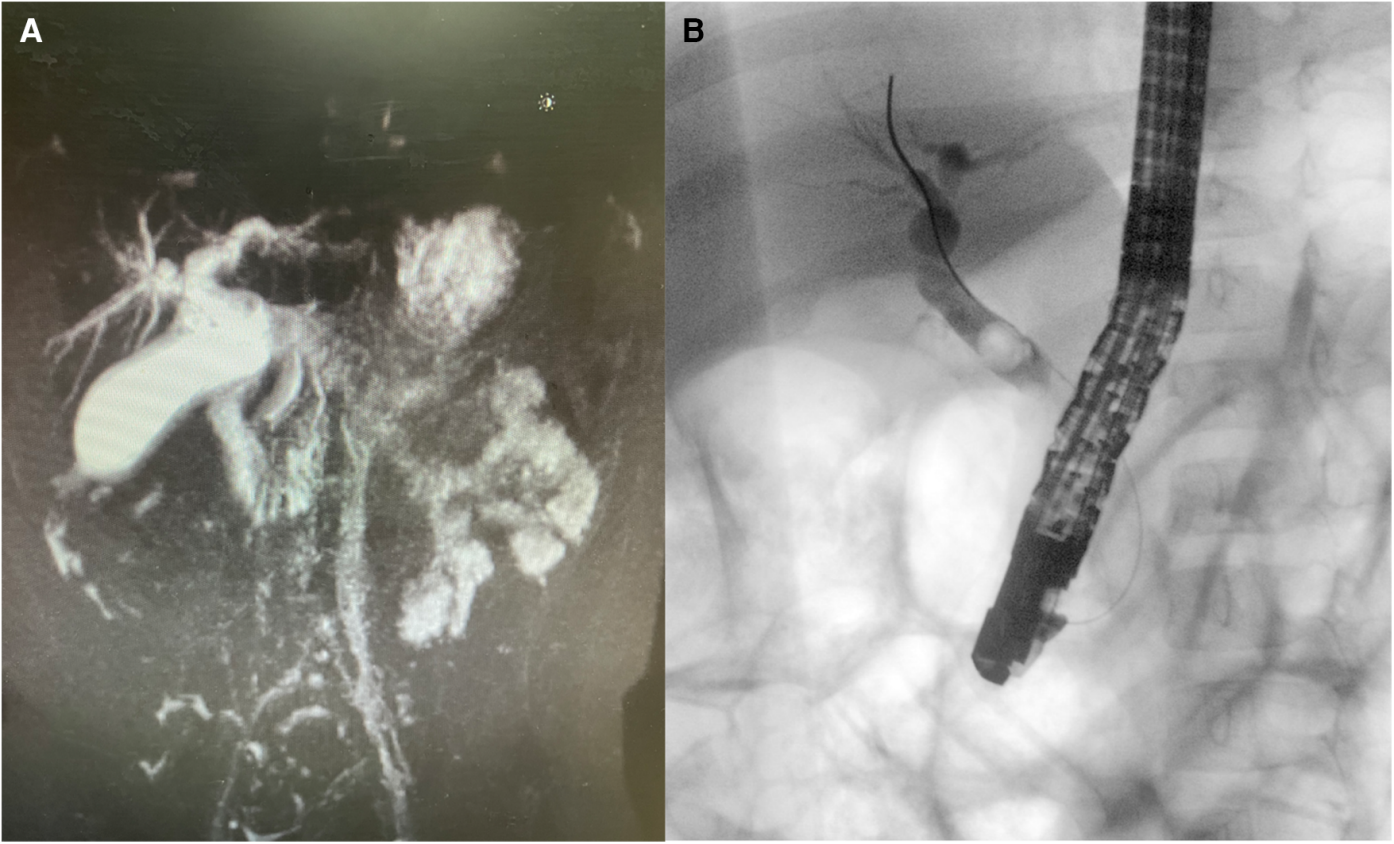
(A) Abdominal CT suggests dilation of the common bile duct and hepatic duct; (B) ERCP revealed a mass in the mid-common bile duct, and a biopsy confirmed it as tumor tissue.
